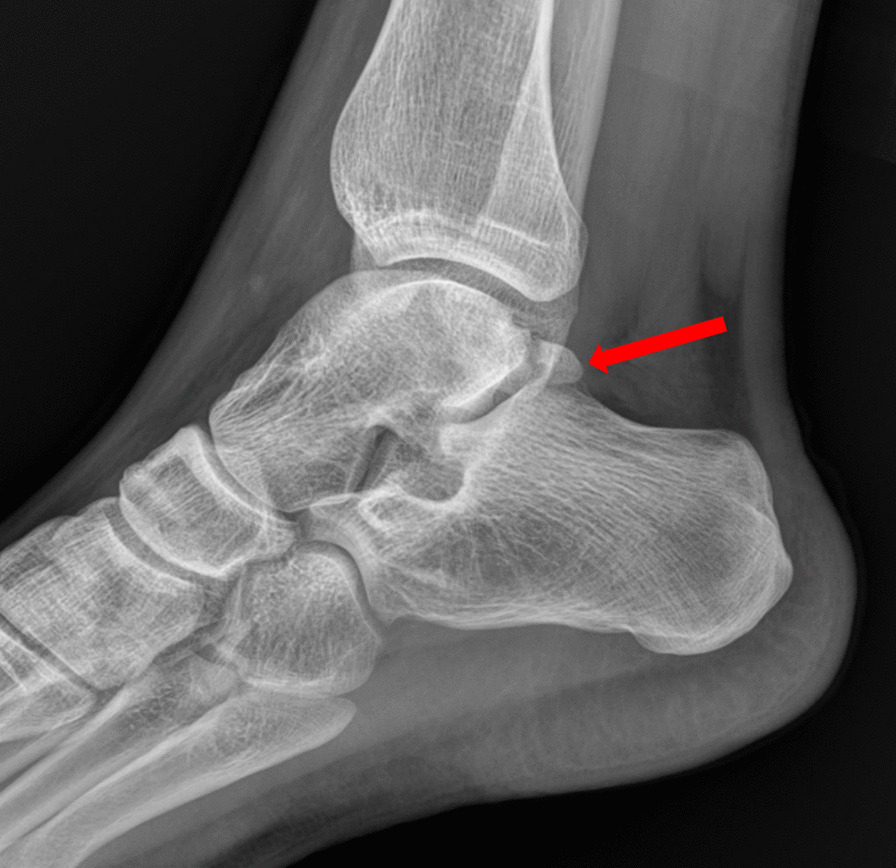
Lateral X-ray showing a posterior lateral tubercle fracture of the posterior process of the talus (Shepherd fracture). The red arrow points to the fracture site
